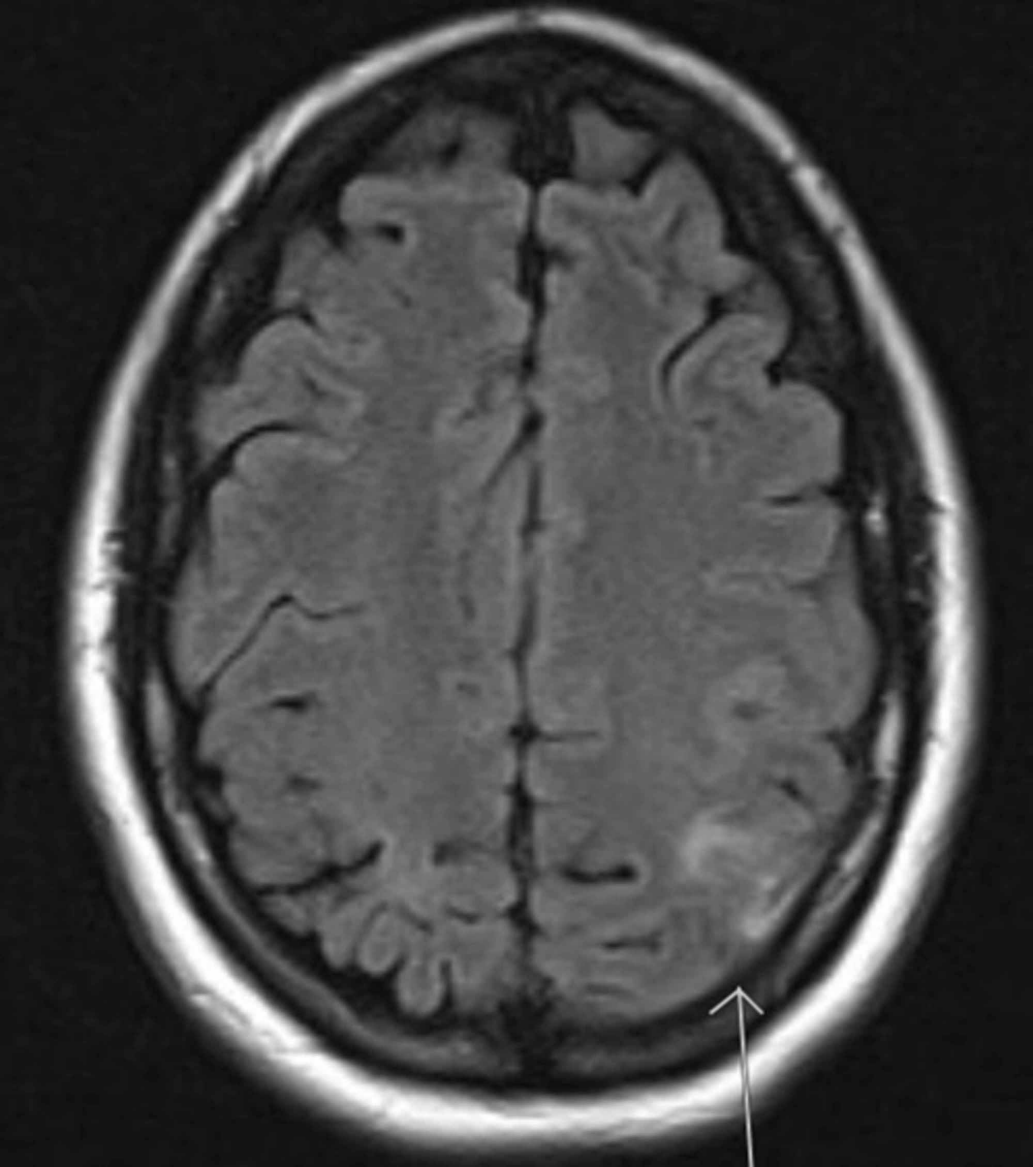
A hyperintense area can be seen on this axial magnetic resonance imaging of the brain (shown with the white arrow)—a prominent sign of cerebral vein thrombosis.
